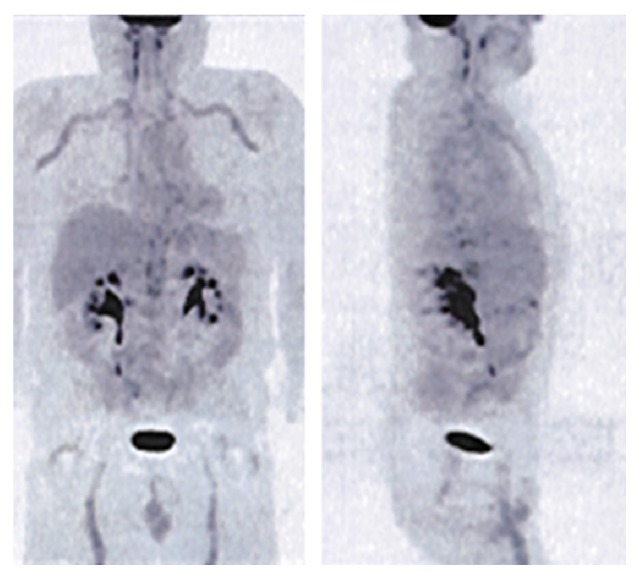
PET scan showing increased uptake of 18F-FDG at the subclavian, carotid, humeral, vertebral, and femoral arteries and less intensively at the ascending and descending aorta, a pattern suggestive of TA. PET: positron emission tomography. 18F-FDG: 18F-fluorodeoxyglucose. TA: Takayasu Arteritis.
